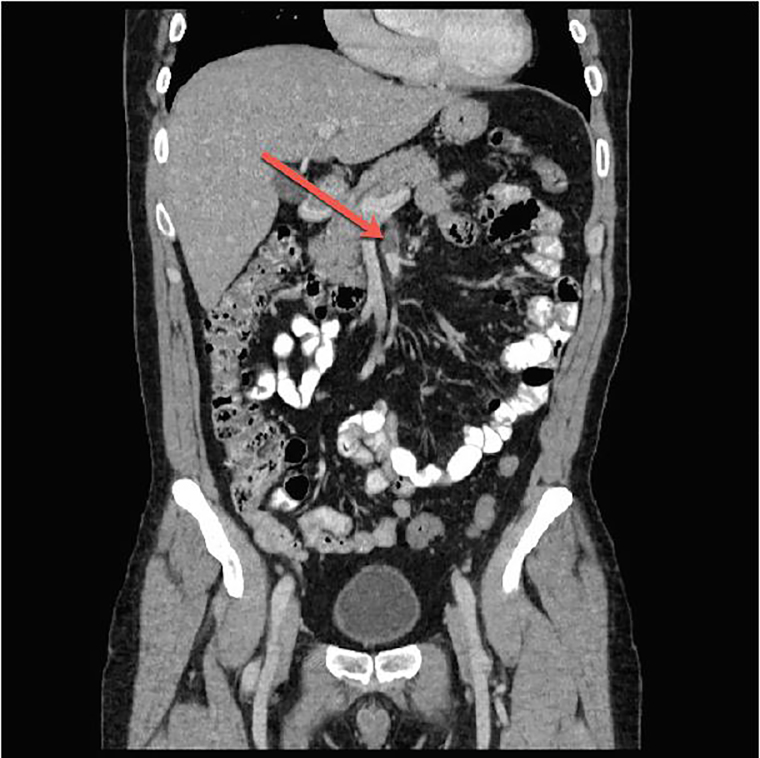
Coronal computed tomography with intravenous contrast demonstrating thrombus (arrow) in the false lumen of the superior mesenteric artery dissection.
